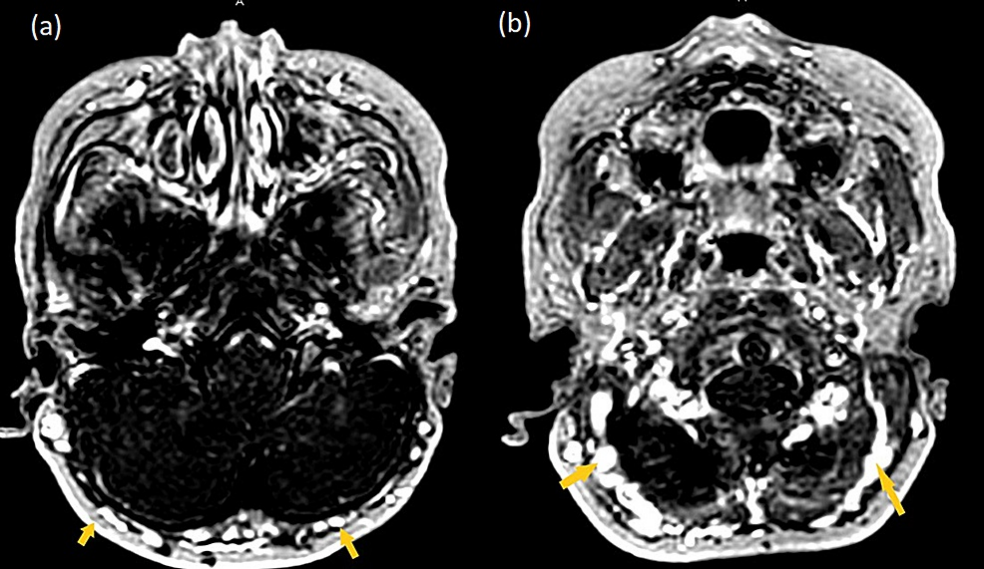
Axial T1W post-contrast images show epicranial varicosities involving bilateral occipital veins (yellow arrows) (a) and paravertebral venous plexus (yellow arrows) (b). T1W: T1-weighted
